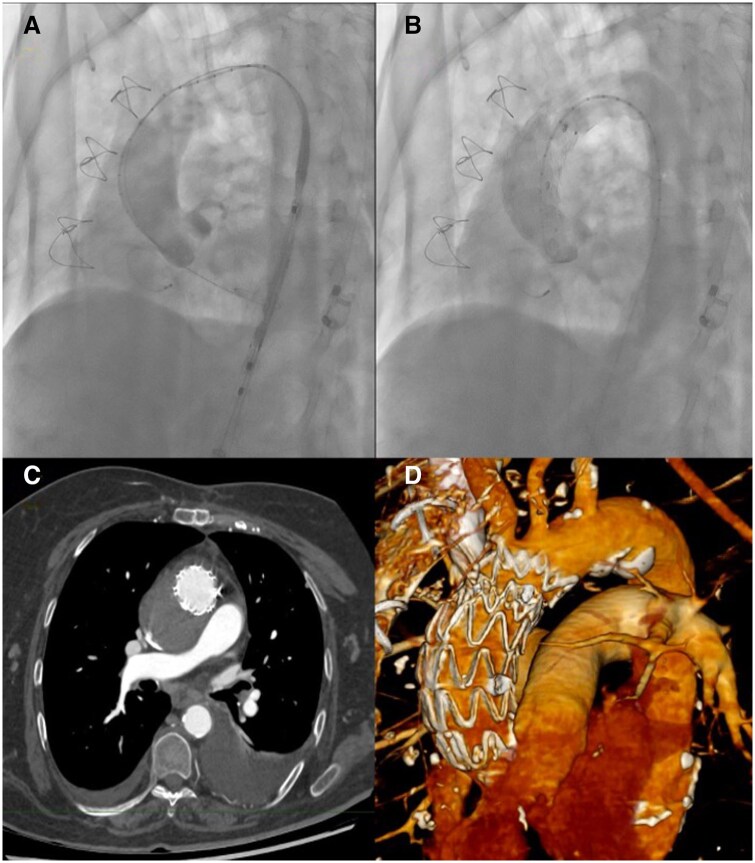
Imaging of Patient no. 5 with a Mycotic Ascending Pseudoaneurysm. (A) Pre-Deployment Angiography. (B) Completion Angiography after Nexus Ascending Module Implantation. (C) Postoperative CTA Confirming Correct Positioning and Preserved Valve and Coronary Flow. (D) 3D Reconstruction Showing Adequate Graft Apposition.
